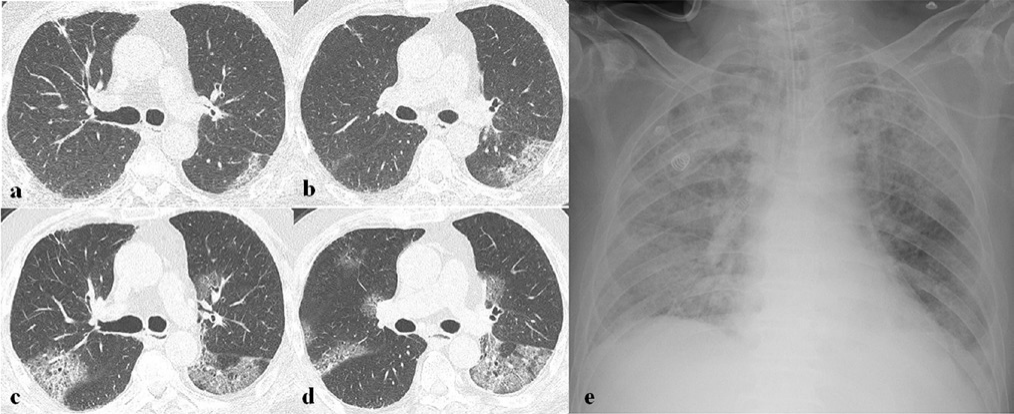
A 78-year-old male patient with SARS-CoV-2 infection. He had a history of exposure to an infected patient and had fever and myalgia for 1 day. (a, b) Initial chest CT scans obtained 2 days after symptom onset show focal subpleural ground-glass opacity (GGO) in left lower lobe. (c, d) Follow-up CT scans obtained 7 days after symptom onset show increased extent of GGO in left lower lobe and new appearance of multifocal subpleural patchy GGOs with the crazy paving appearance in both lungs. (e) Follow-up chest radiograph obtained 15 days after symptom onset shows bilateral diffuse GGOs and consolidation in both lungs, suggesting diffuse alveolar damage. He died of respiratory failure.
